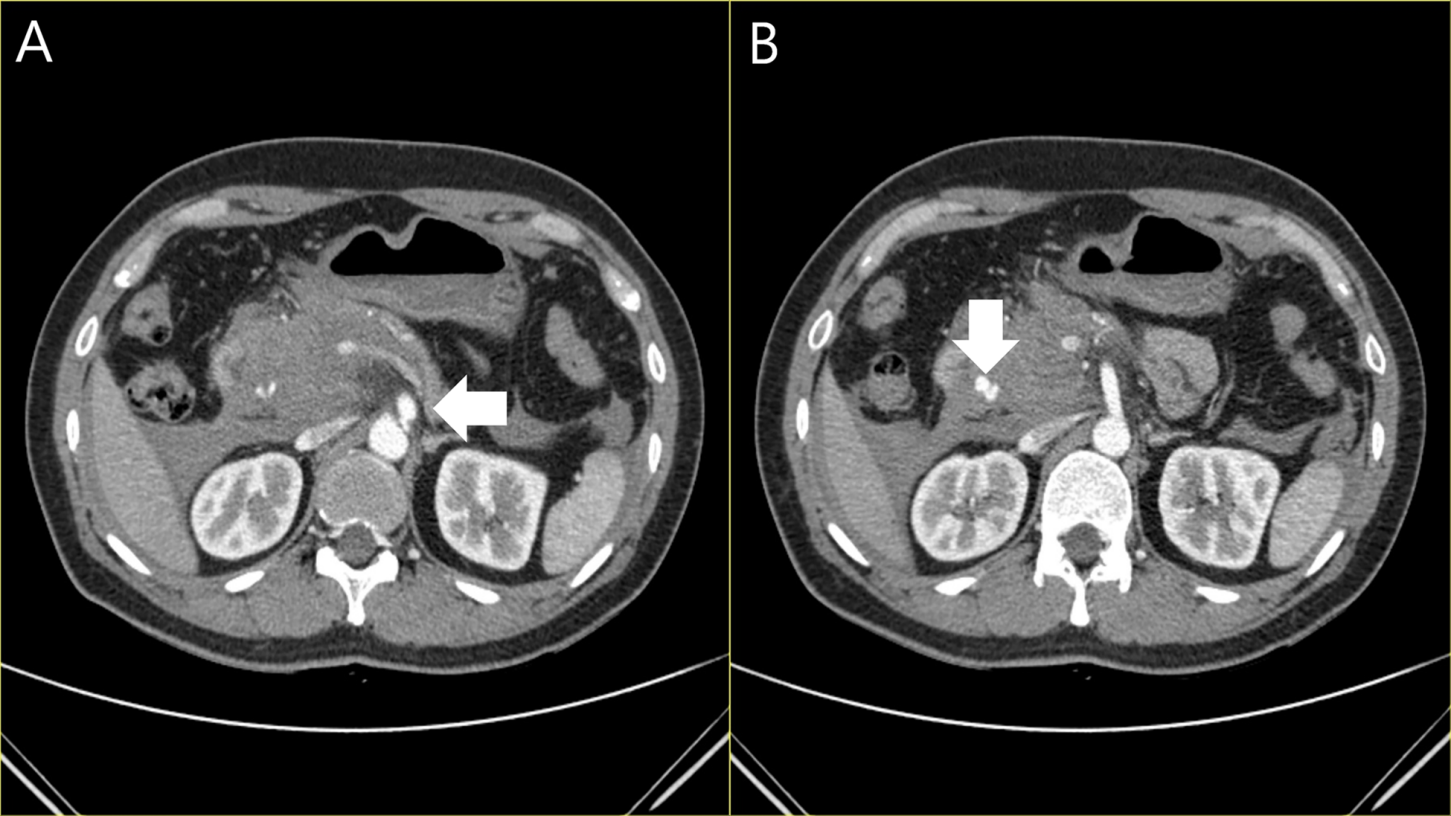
Initial CT scans (patient No. 8): (A) celiac axis arterial dissection and (B) pseudoaneurysm of gastroduodenal artery.
